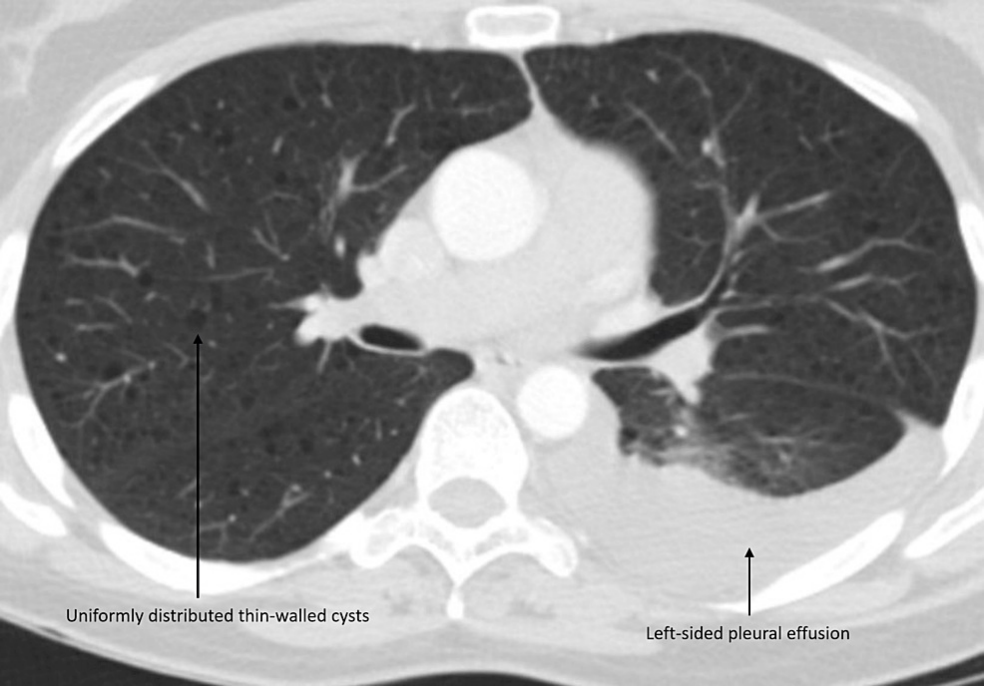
CT chest demonstrating diffusely distributed thin-walled cysts in both lungs with moderate left pleural effusion.
